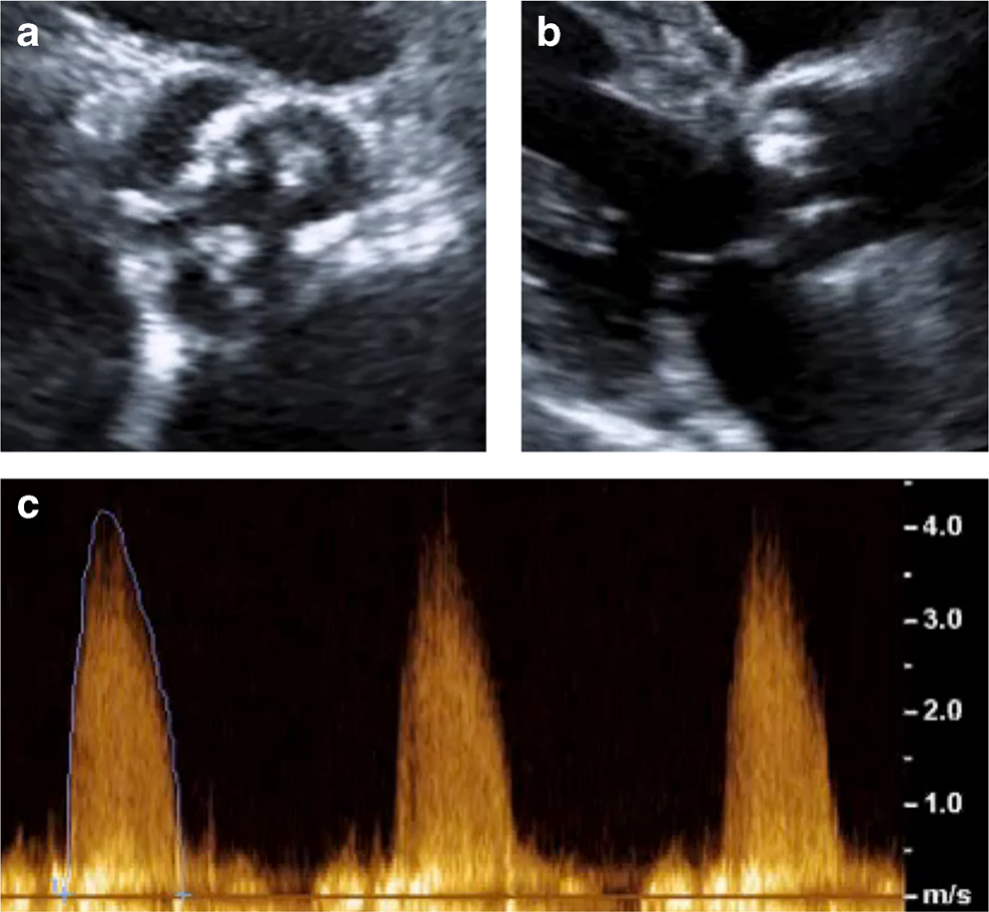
Echocardiographic assessment of a patient with severe aortic stenosis. a Short axis view showing heavily calcified leaflets. b Parasternal long axis view showing large calcium deposit on right coronary cusp with restricted valve opening. c Right sternal edge continuous-wave Doppler with aortic valve velocity >4 m/s, corresponding with severe stenosis
